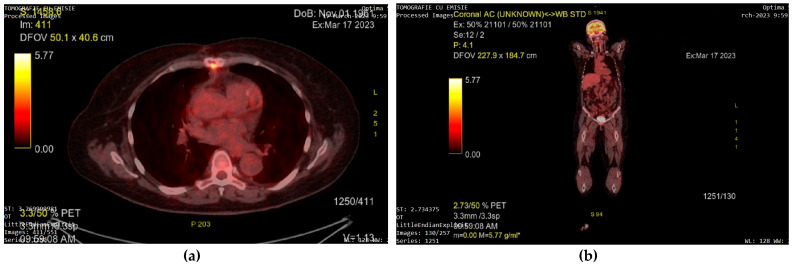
18F-FDG fluorodeoxyglucose (18F-FDG) PET-CT showing: (a) an encapsulated effusion adjacent to the right ventricle infundibulum (white circle) with minimum calcification, thin minimally active margins, and no other 18F-FDG fixation zones at this level; slight 18F-FDG fixation at the sternum level due to recent sternoraphy; (b) no distant metastases on the whole-body examination.
